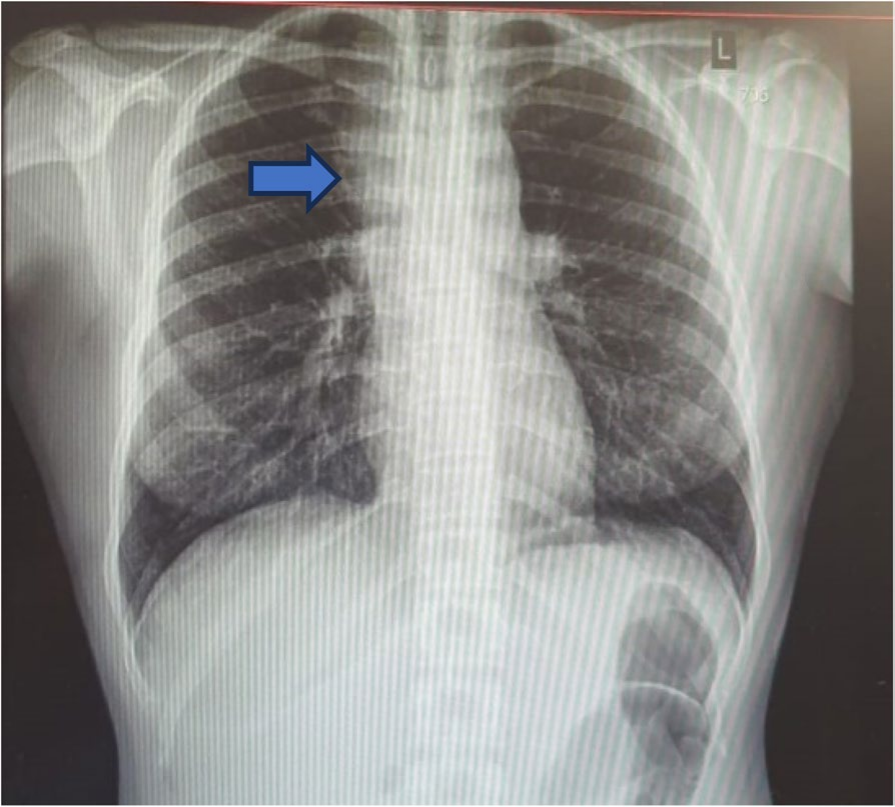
Chest X-Ray showing widened mediastinum (blue arrow)
